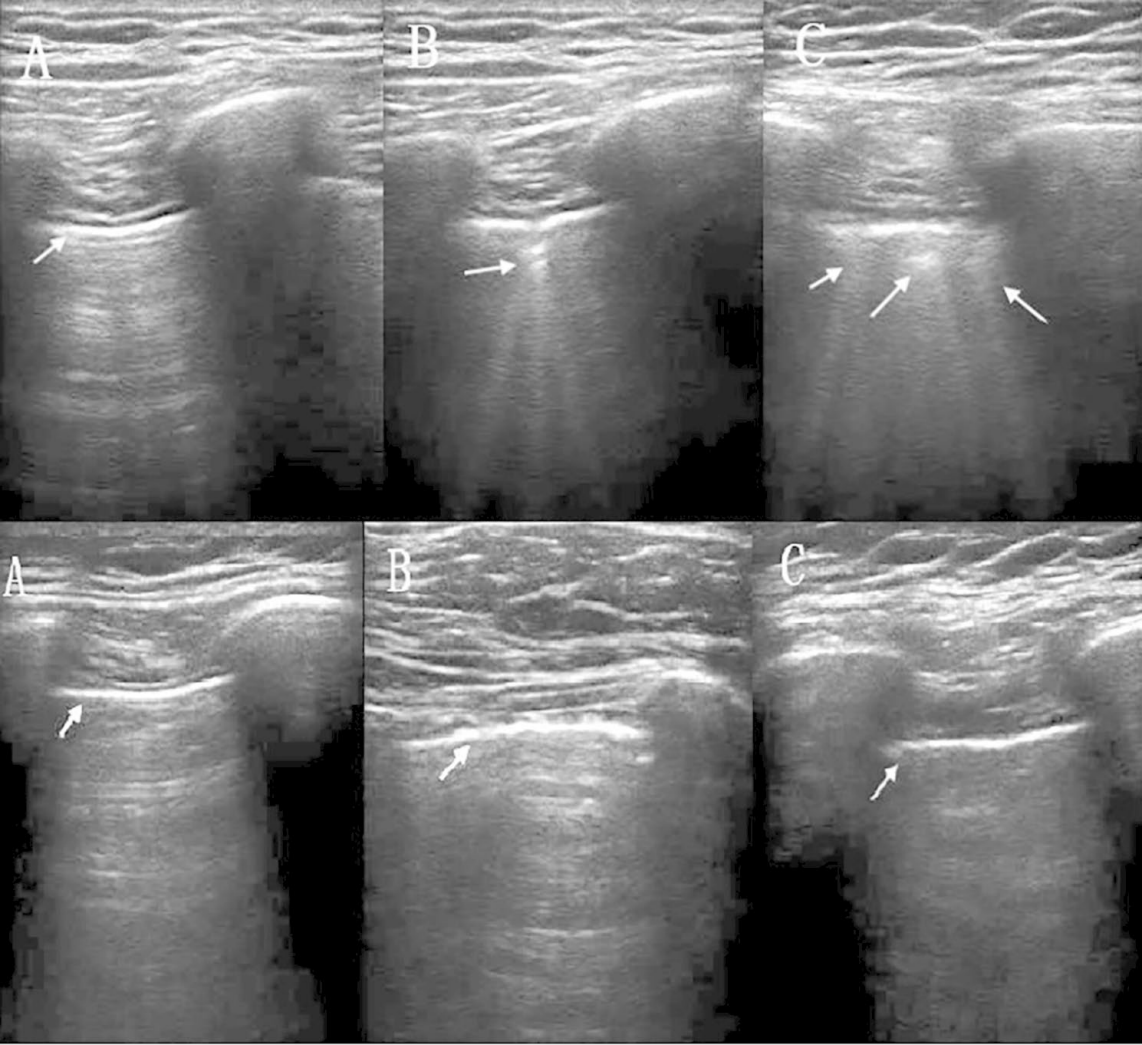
Representative lung ultrasonography images at different time points The first group is the control group, and the second group is the titration group. (A) Preinduction. (B) Immediately after extubation. (C) One hour after extubation. At least three hyperechoic B-lines can be seen originating from the pleural line in the control group either immediately or 1 h after extubation
